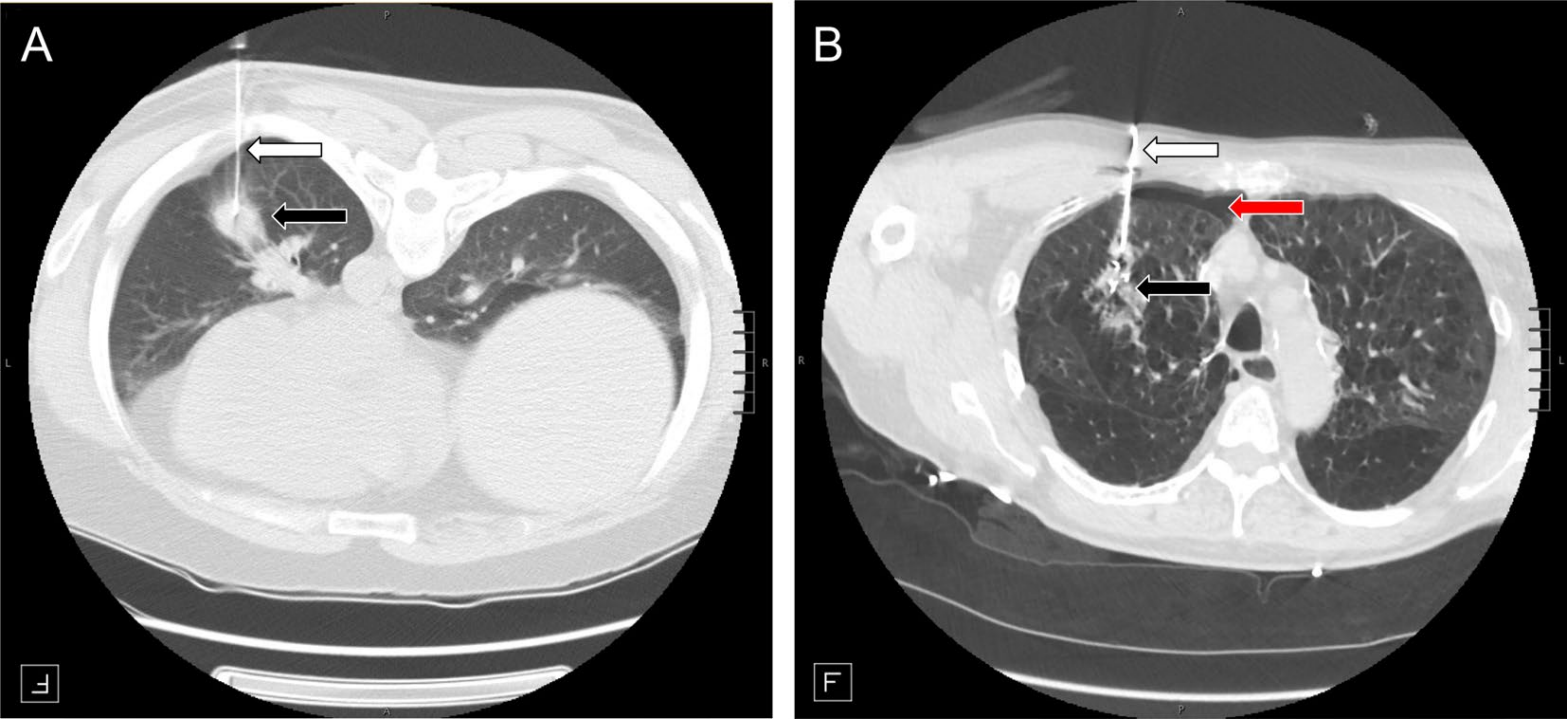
Example cases. CT scans from CT-guided transthoracic lung biopsy in two patients. Biopsy needle (white arrow) and the nodule biopsied (black arrow) are shown. A) Patient is a male in their 40s presenting with no emphysema (as determined by quantitative emphysema algorithm), lying in the prone position, with a nodule in the left lower lobe. Our risk nomogram and calculator predicted this patient to have low risk of pneumothorax requiring chest tube (1.86% [1.13% - 3.13%]). The patient post biopsy had a tiny pneumothorax and did not need chest tube placement. B) Patient is a male in their 60s with severe emphysema (as determined by quantitative emphysema algorithm), lying in the supine position, and with a nodule in the right middle lobe. Our risk nomogram and calculator predicted this patient to have high risk (14.70% [10.90% - 19.50%]). The patient developed intraprocedural pneumothorax (red arrow) which grew into a large pneumothorax and chest pain necessitating a chest tube placement
